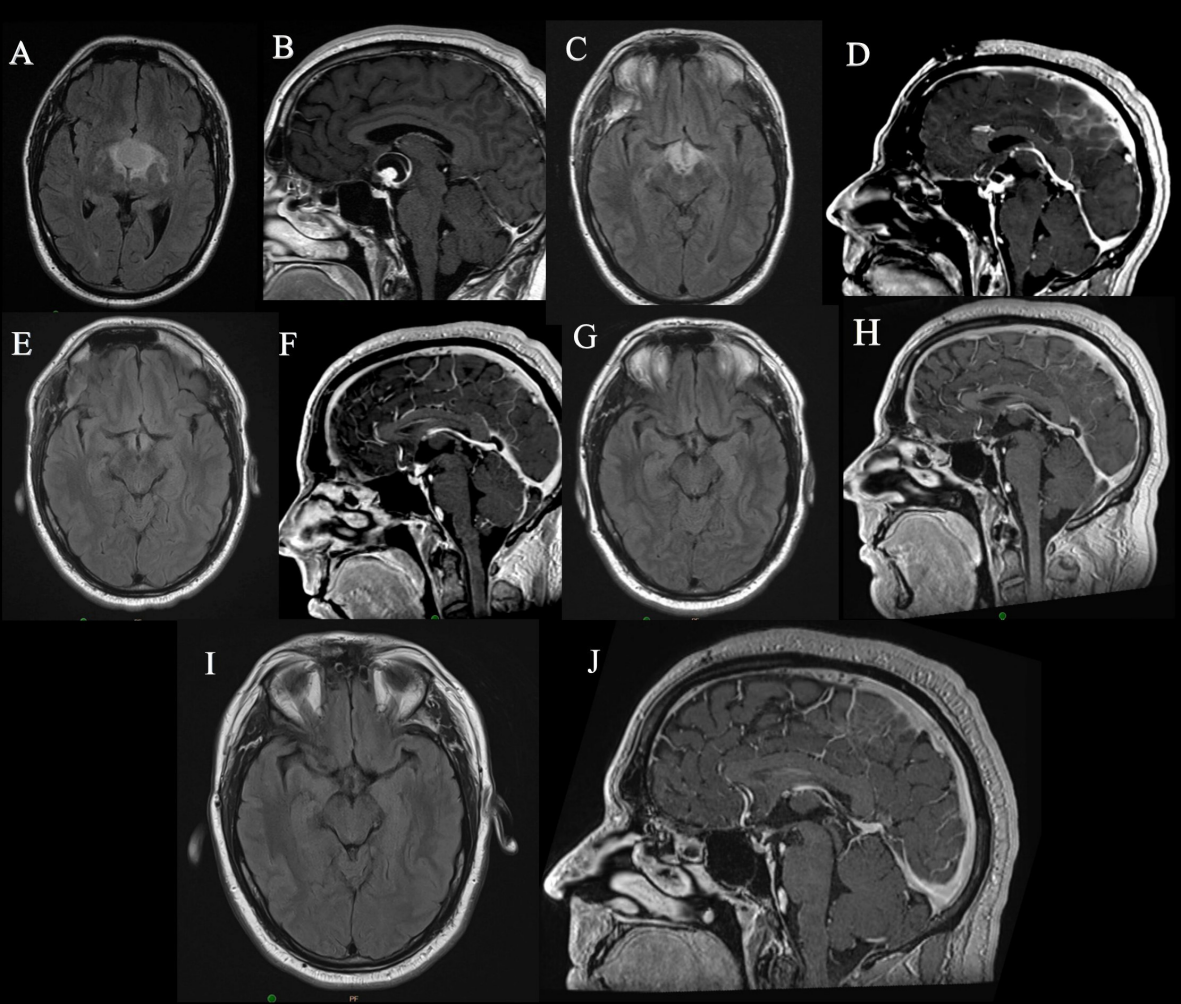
MRI images of Case 1: (A, B) Preoperative MRI images, (C, D) Immediate postoperative MRI images showing residual tumor, (E, F) MRI images after 1 month of dabrafenib, trametinib therapy showing significant reduction in size of tumor, (G, H) MRI images after 2 years of therapy, (I, J) MRI images obtained in last follow up, 5 years since surgery and 3 years since cessation of therapy.
